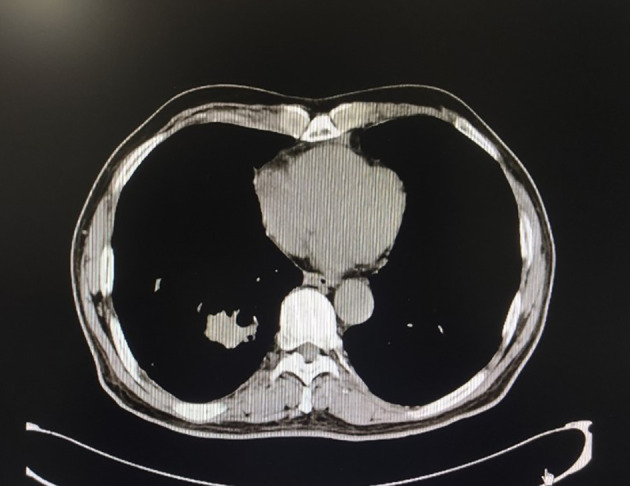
Chest CT one month after surgery.
